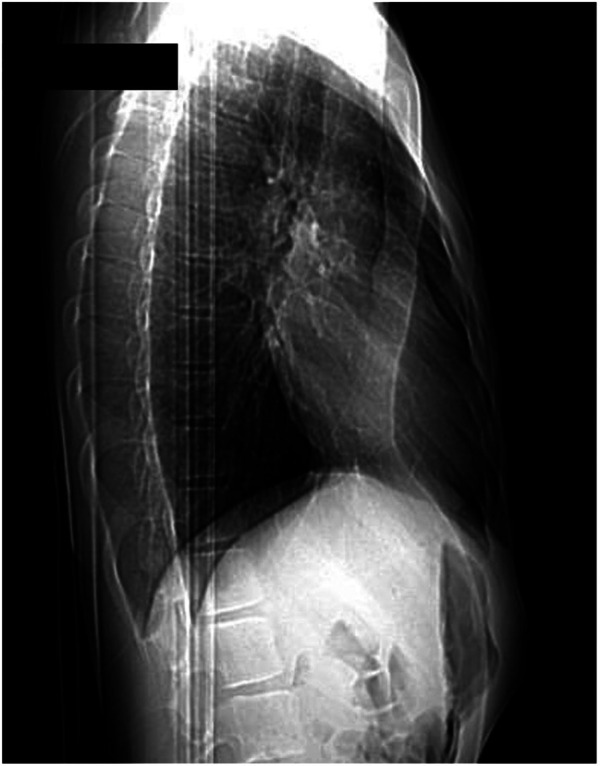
Pre-operative chest x-ray to measure the thickness of the sternum.
